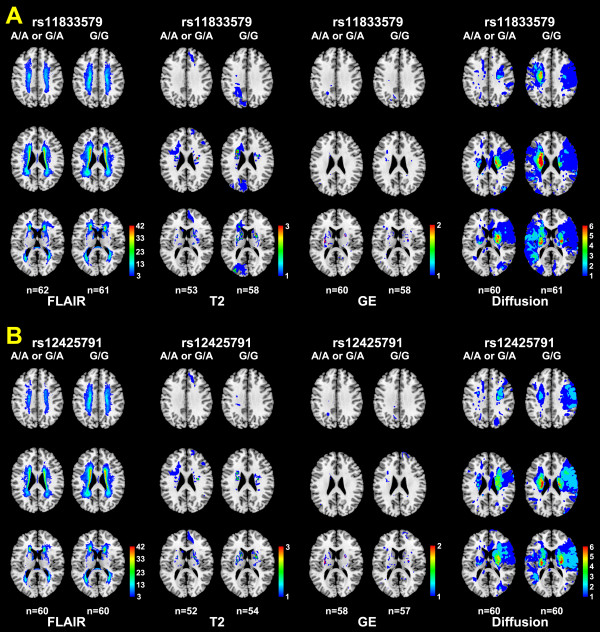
Accumulation MR lesion maps depending on the rs11833579 (A) or rs12425791 (B) genotype. Quantitative maps of diffusion/fluid attenuation inversion recovery (FLAIR)/T2/gradient-echo (GE) MR images show no significant difference between the age-sex matched patients with the rs11833579 A/A or G/A genotype and those with the G/G genotype (A). In the age-sex matched patients with the rs12425791 A/A or G/A genotype (B), compared with the ones with the G/G genotype, the extent of leukoaraiosis in the centrum semiovale (first row) and corona radiata (second row) on FLAIR MRIs appears to be smaller. The pseudocolor bars indicate numbers of subjects with lesions overlapping a specific pixel.
